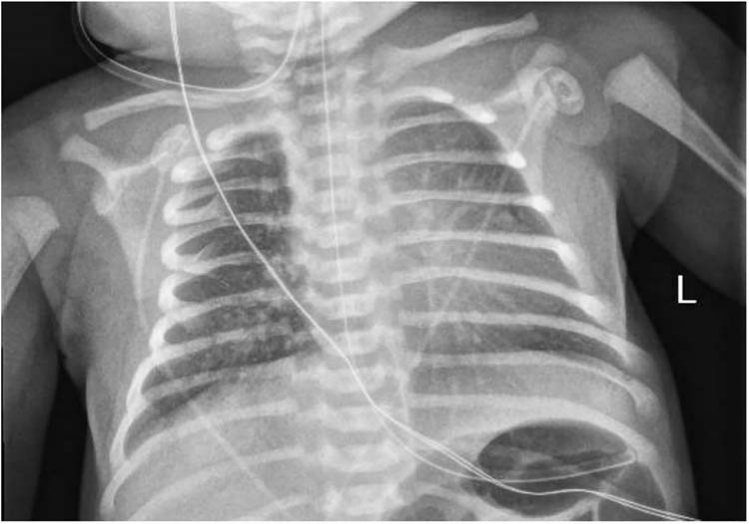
Chest x-ray with right medial lung opacity.
